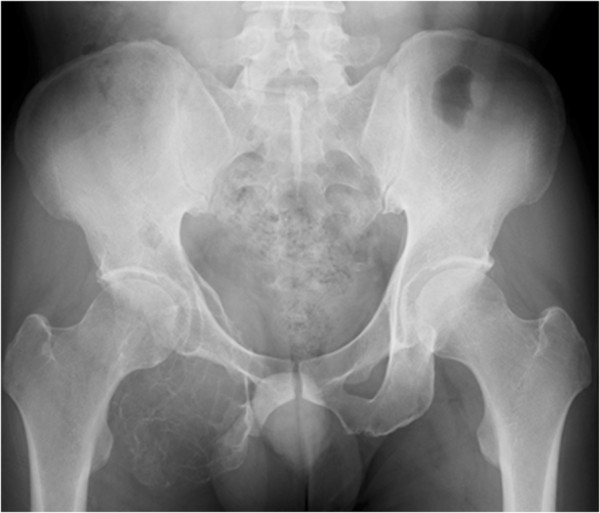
X-ray showed a tumor of the right ischium with thinning of the cortical bone.
